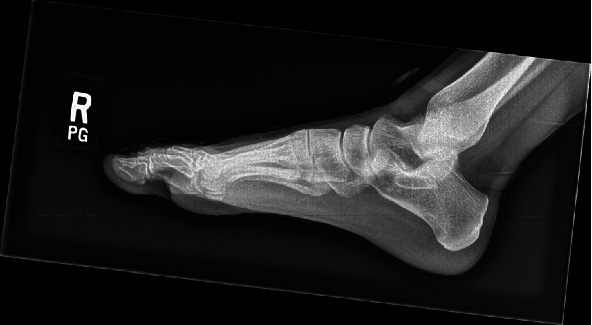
Lateral radiograph of the right foot taken three years post-OCA implantation with complete resolution of hallux rigidus.
